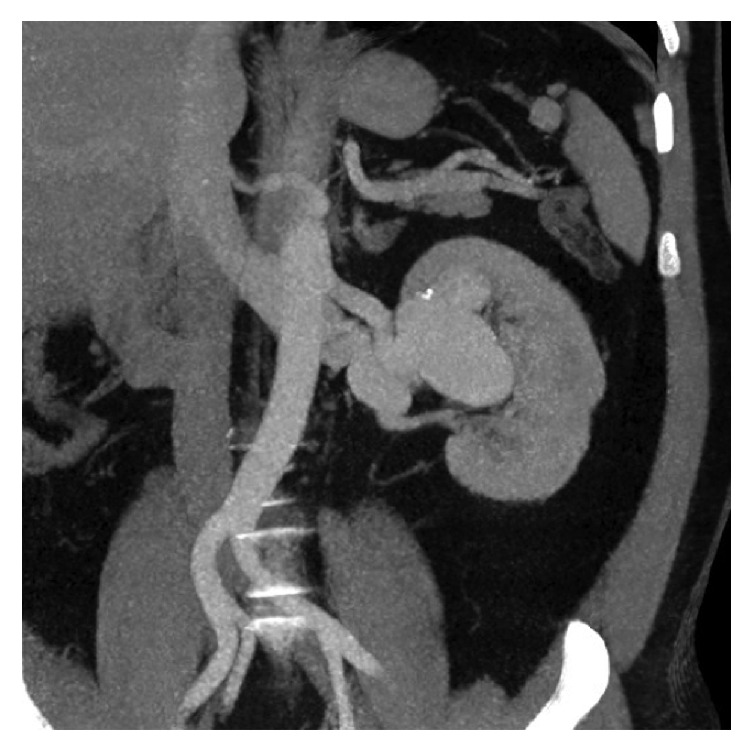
Admission CT-scan showing complex vascular intraparenchymal renal anomaly.
